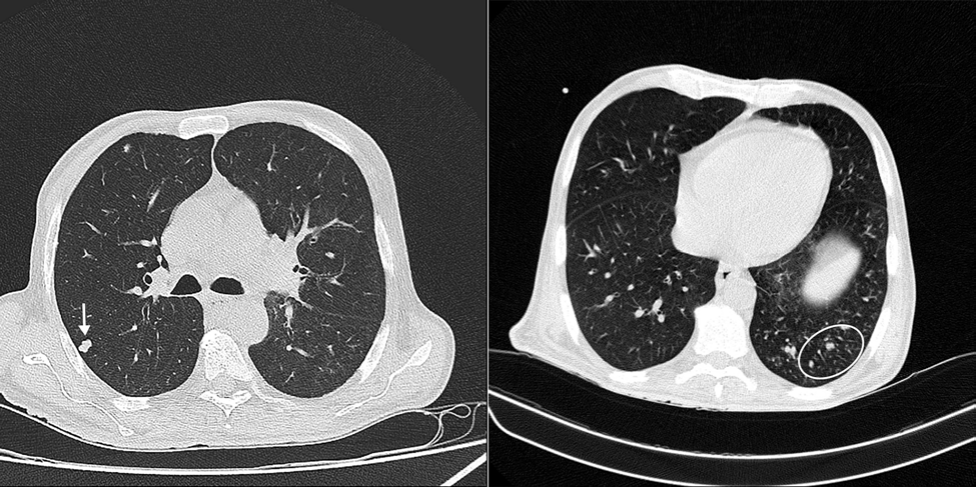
Pulmonary nodule (white arrow) and tree-in-bud pattern (circled area) in the chest CT scan
